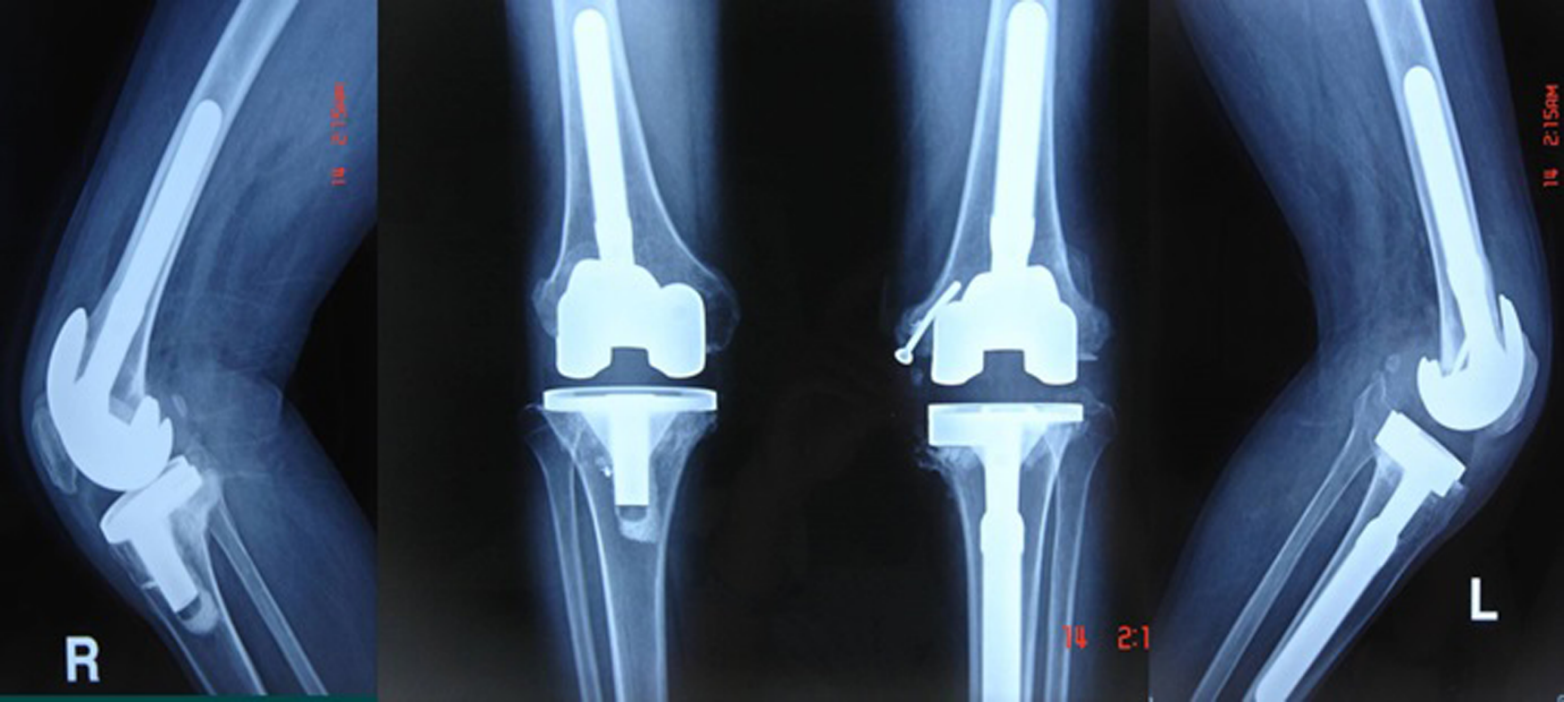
Radiograph of the anterior and lateral post-TKA positions after TKA, indicating that the position of the prosthesis was favorable.
